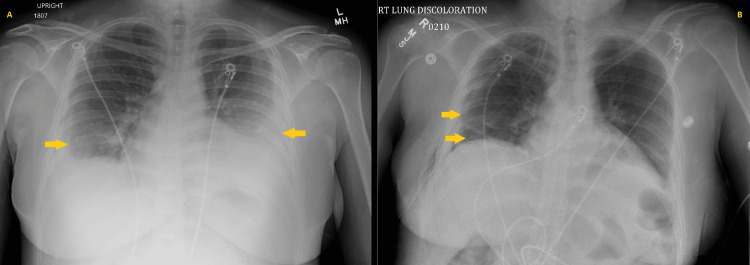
Chest radiographs obtained on readmission one month after the initial hospitalization showed worsening bilateral pleural effusions compared to day 9 of rehospitalization (A) and following right-sided decortication with placement of two chest tubes (B).
